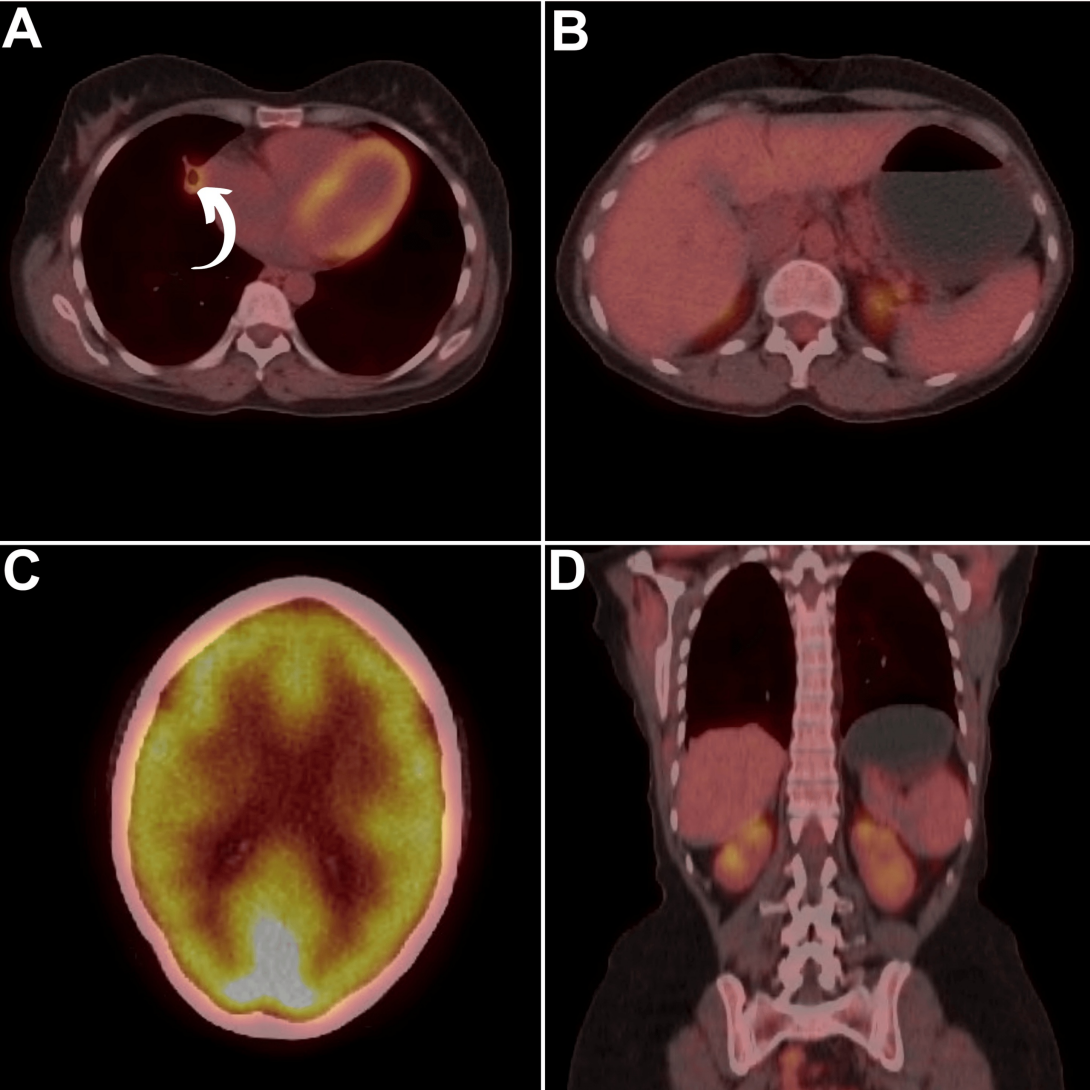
Follow-up fluorodeoxyglucose PET/CT (A) axial lung PET/CT, (B) axial hepatic PET/CT, (C) axial brain PET/CT, and (D) coronal PET/CT revealed evidence of complete metastatic response and a marked primary tumor reduction (curved arrow). PET/CT, positron emission tomography/computed tomography
